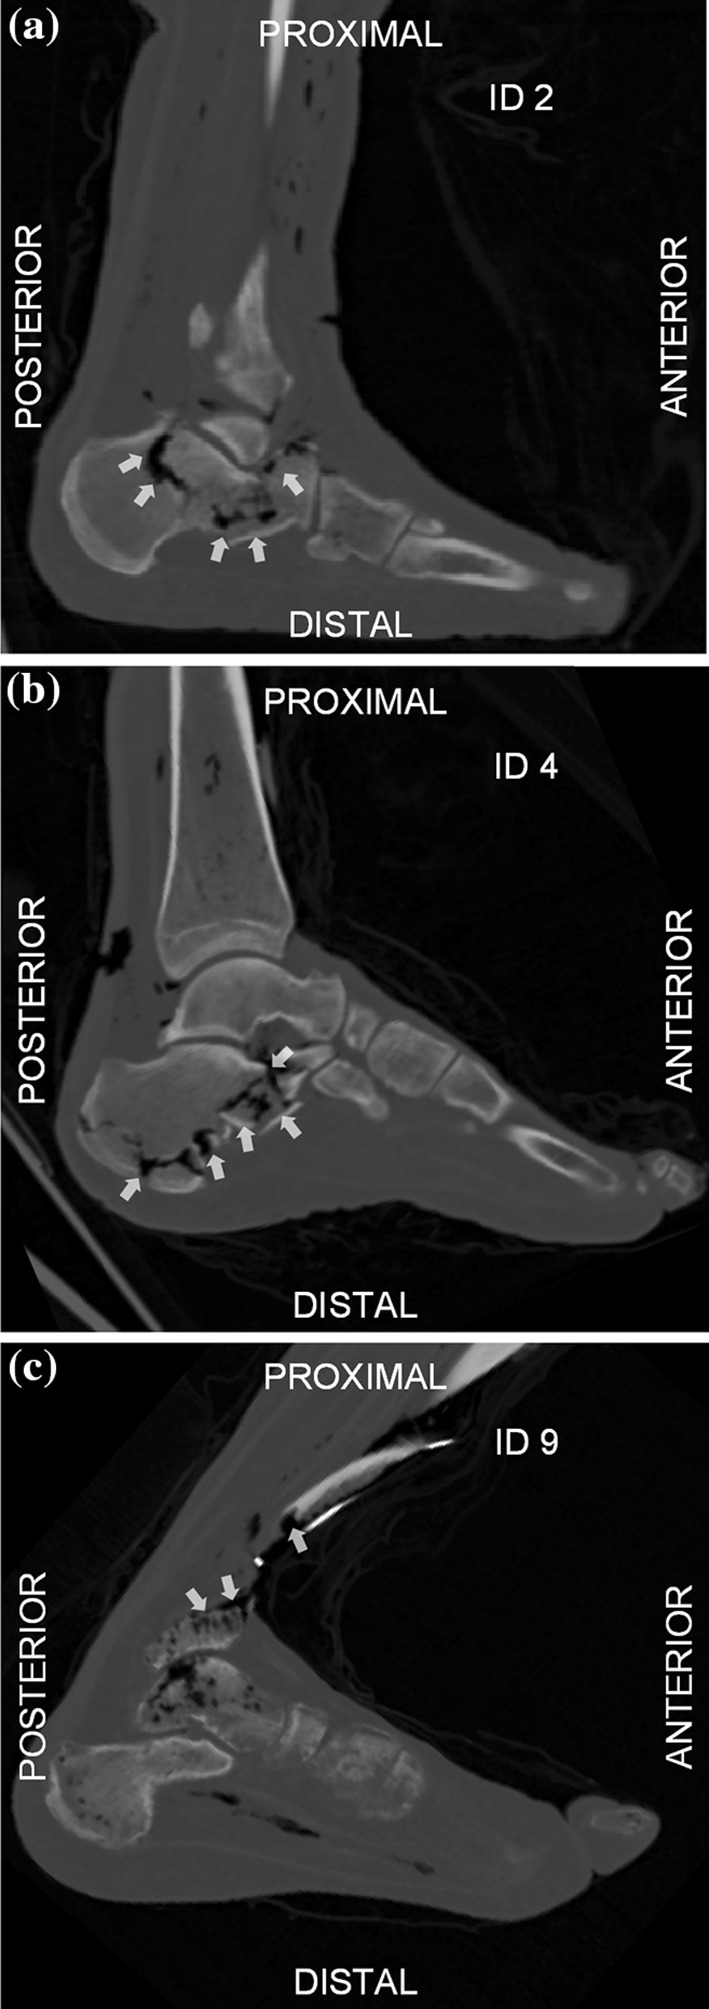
Sagittal CT scans showing fractures (white arrows) in PMHS lower limbs tested when set at posture (a) 90°–90°, (b) 120°–90°, and (c) 120°-120°.
